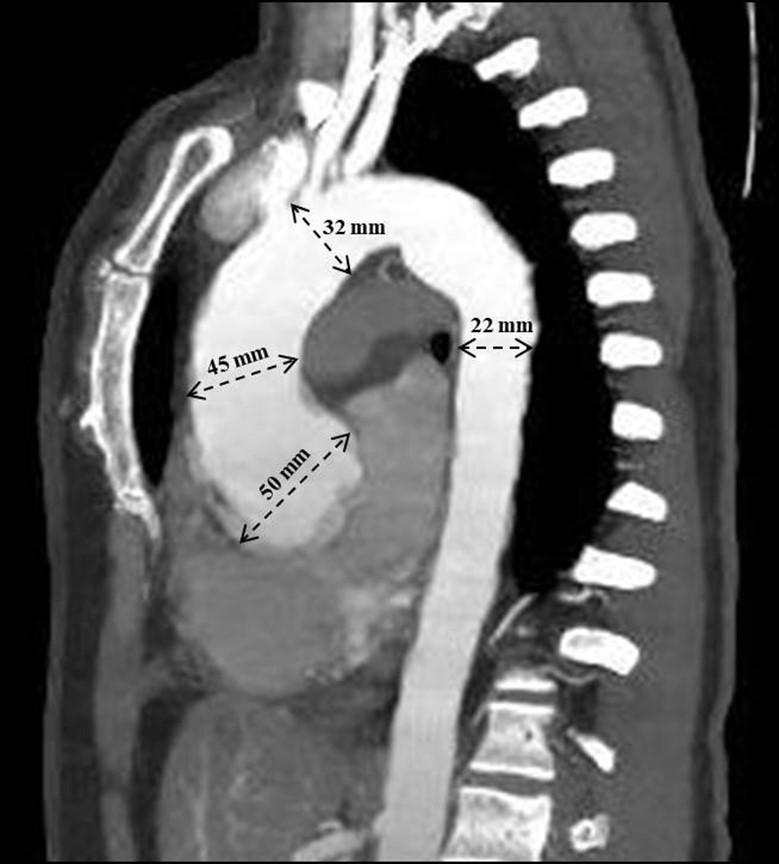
Contrast-enhanced chest CT angiography showing dilatation of the aortic root with a diameter greater than 50 mm in the ascending segment, the ascending segment 45 mm, the aortic arch 32 mm and the descending segment 22 mm.
